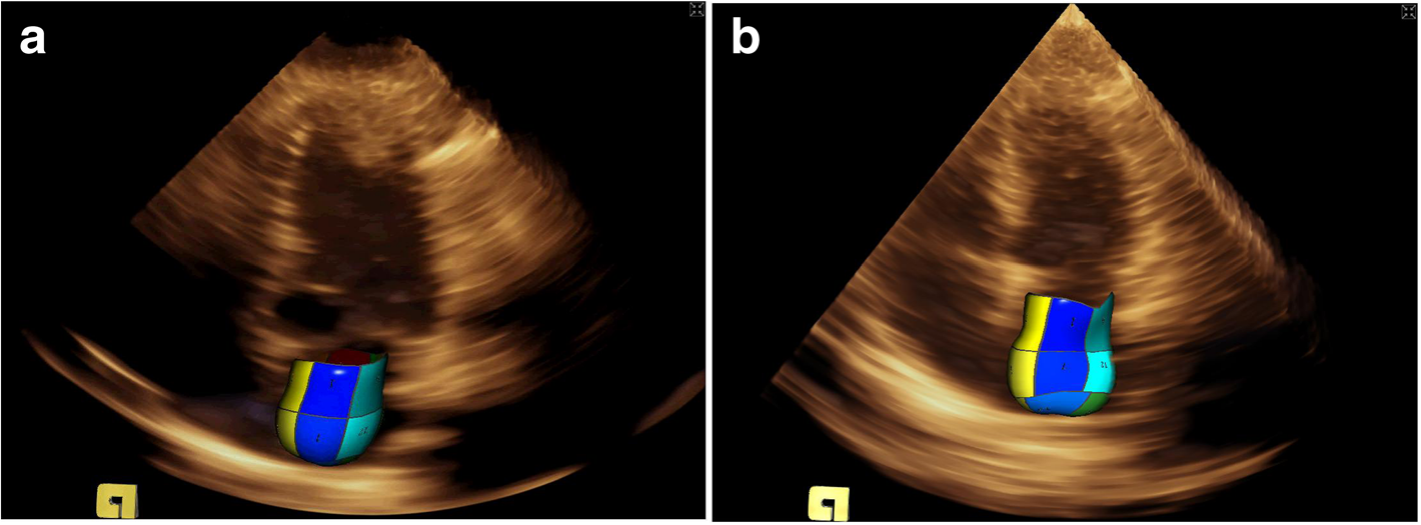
Real-time three-dimensional echocardiography (RT-3DE) images of left atrial volume of healthy controls (a) and patient cases (b)
